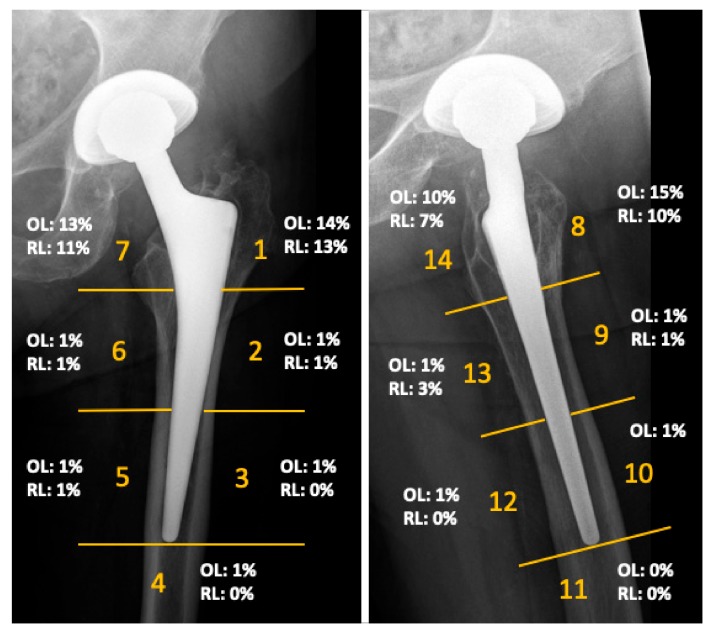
Results of the radiographic evaluation showing the distribution of radiolucent lines (RL) and osteolysis (OL), as seen on anteroposterior and lateral radiographs according to Gruen zones at a mean follow-up of 14 years.
